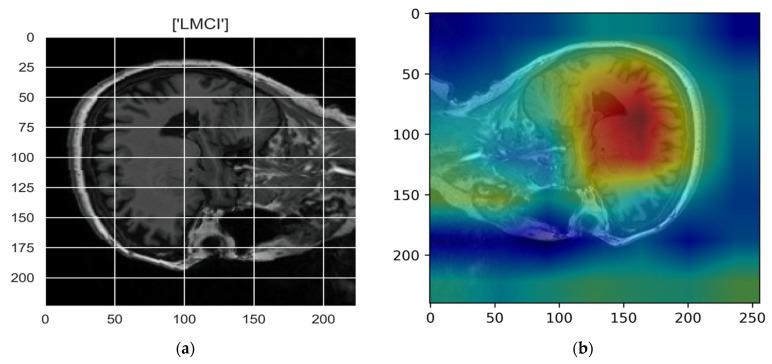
Grad-CAM explanation for the prediction of class LMCI: (a) MRI image, (b) Grad-CAM attention map.
